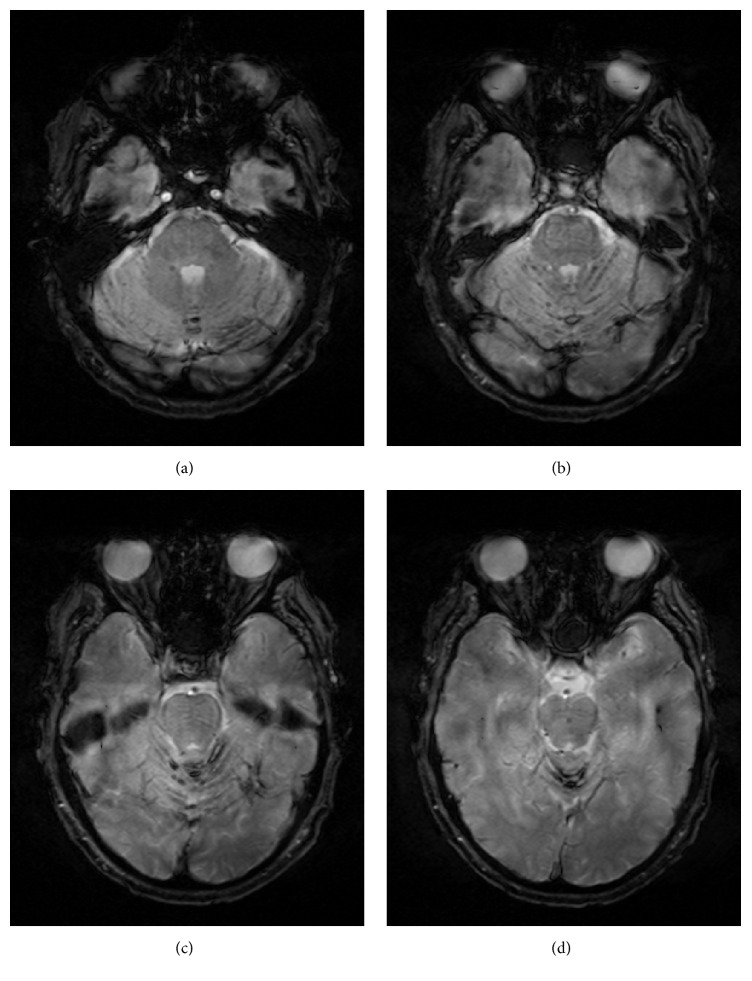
T2-weighted gradient ECHO MRI of the brain: superficial siderosis with hemosiderin deposition seen in the vermis and folia of the cerebellum.
